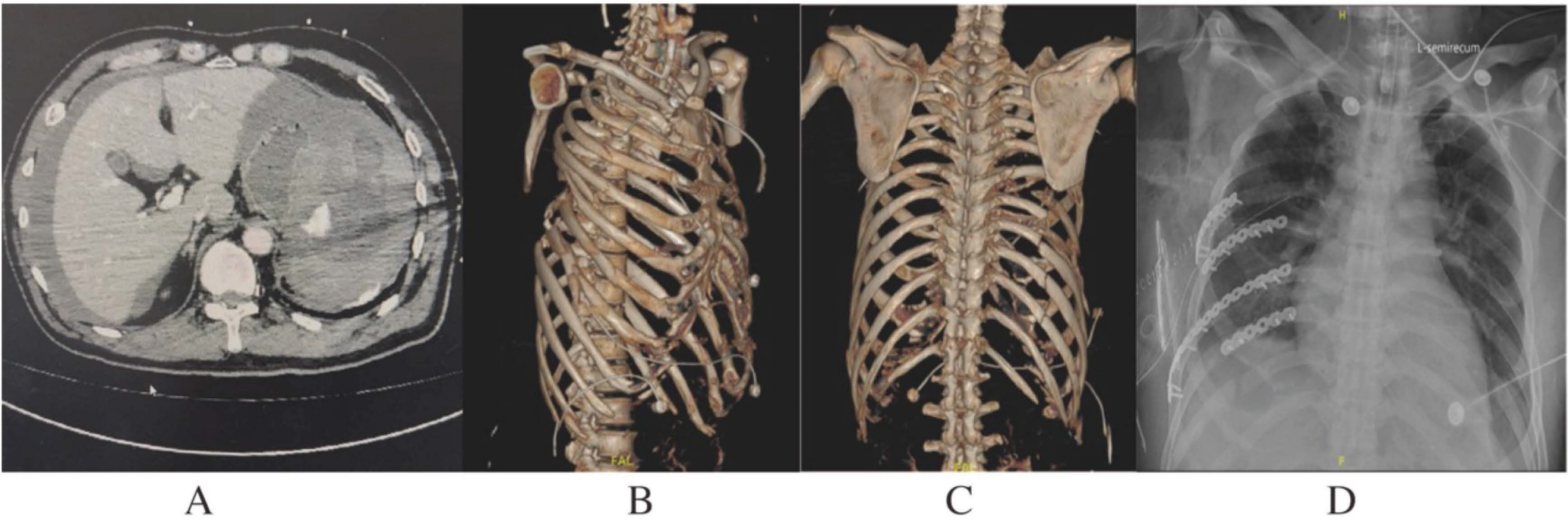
A Polytrauma Patient with Splenic Rupture and Multiple Rib Fractures. A 52-year-old male sustained fall-from-height injury with an ISS of 25, diagnosed with hemorrhagic shock, splenic rupture, and multiple rib fractures. A, contrast-enhanced CT scanning revealed a Grade V splenic rupture with contrast extravasation. B, C, the 3rd to 10th rib fractures with severe chest wall deformity of right side. On admission, the patient underwent an emergency exploratory laparotomy and splenectomy and was transferred to the ICU for resuscitation. D, 20 hours after successful resuscitation, partial ribs (5th to 8th) were fixed to restore the relative stability of the chest.
